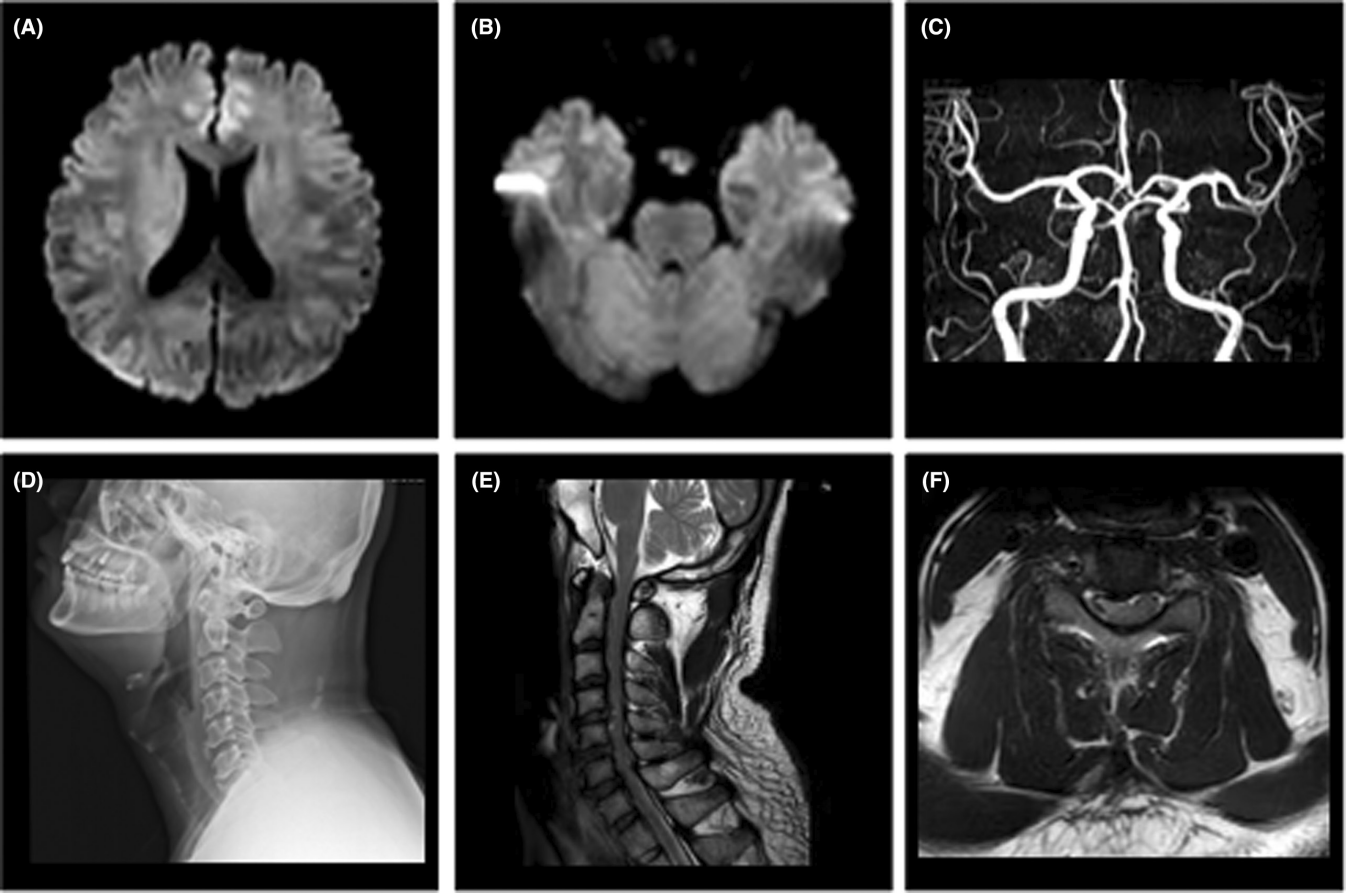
Brain MRI and MRA images and cervical spine X‐ray and MRI. MRI showed no diffusion restriction in the brain (A, B). MRA showed no obvious stenosis or occlusion (C). X‐ray examination revealed that cervical vertebral physiological curvature became straight (D). Cervical spine MRI revealed a patchy T2 high signal that can be seen in the cervical spinal cord at the C4/5 level in both sagittal and axial positions with intervertebral disc prolapse (E, F).
